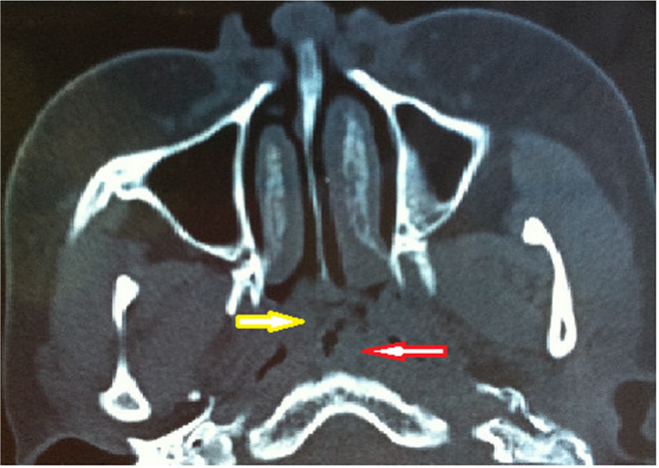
Preoperative CT findings of the patient. Overlapping image of ectopic PTC (yellow arrow) and hypertrophic adenoid (red arrow) in nasopharynx.
